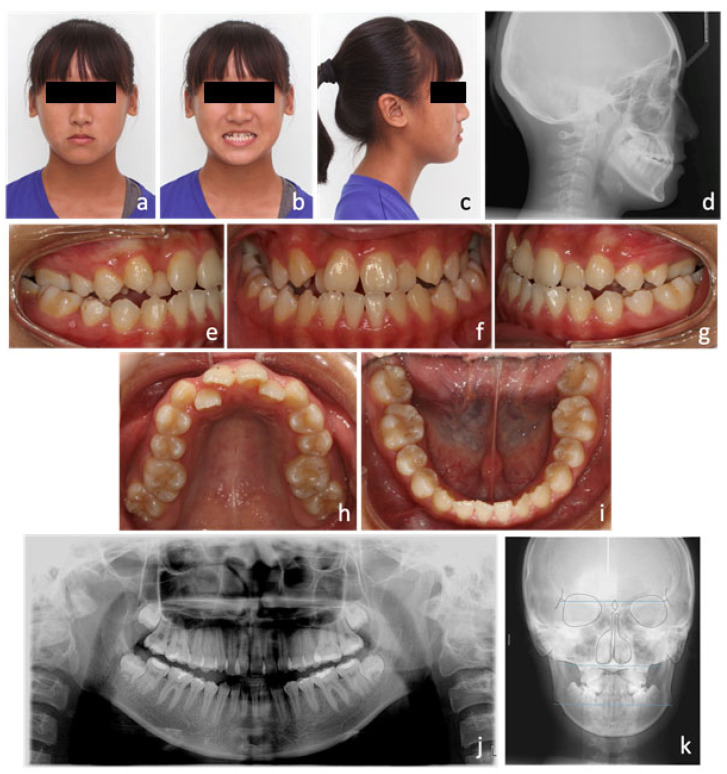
Initial photos and radiographs. (a) Extra-oral photos showed flat midface and mild facial asymmetry with chin deviation to the right. (b) Vertical facial height proportion was balanced but the upper incisor show was minimal. (c) Lateral profile showed a mild concave profile. (d) Skeletal pattern was class III with an average Frankfort-mandibular plane angle from the cephalometric radiograph. (e–i) Intra-oral photos showed moderate crowding (5.4 mm deficit) in the upper arch, mild crowding (1.9 mm deficit) in the lower arch, and bilateral crossbites in the buccal segments. (j) The initial orthopantographic (OPG) radiograph. (k) Maxillomandibular transverse discrepancy was 35.5 mm measured from Ricketts Rocky Mountain analysis.
